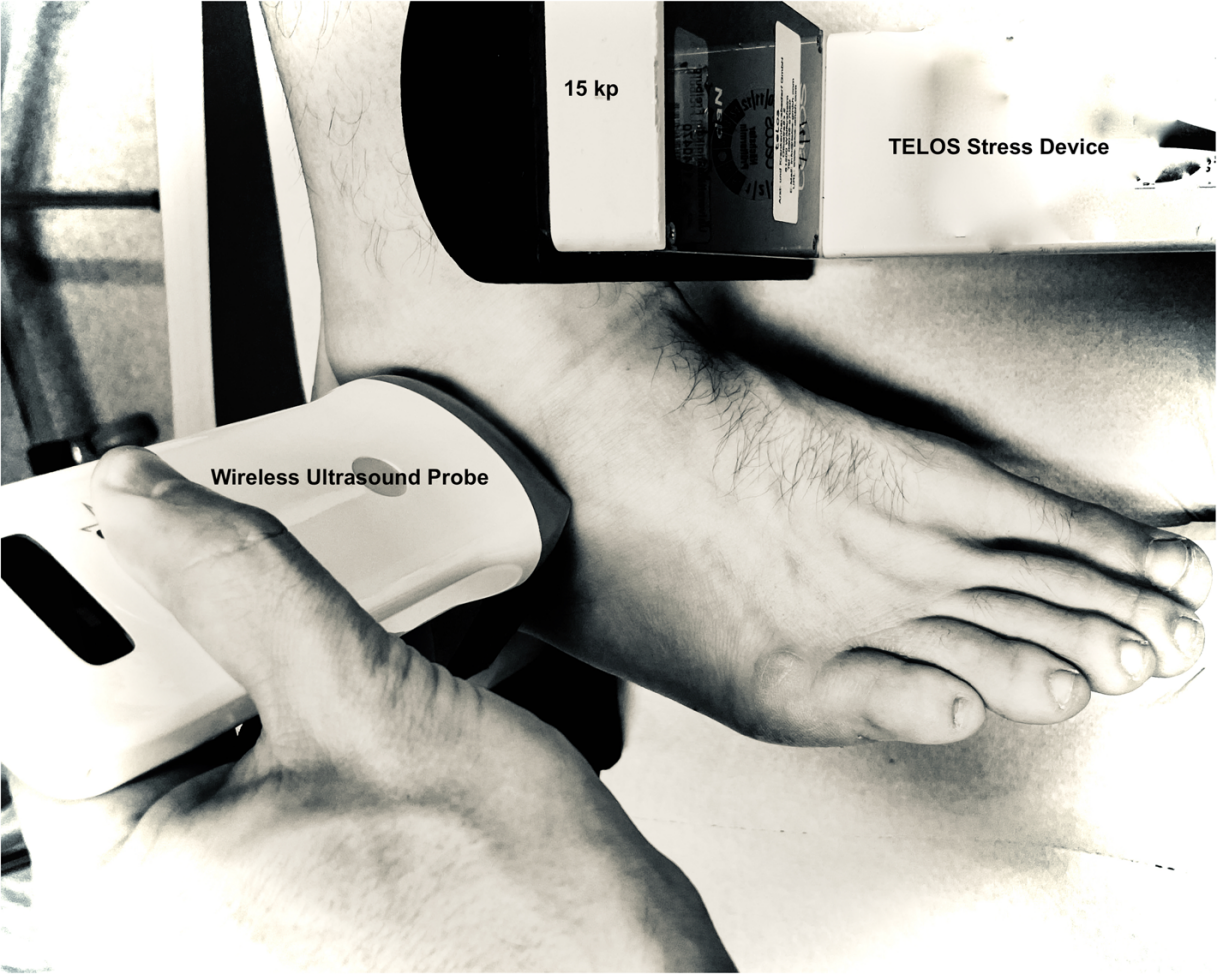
Setup for the stress ultrasound measurement
